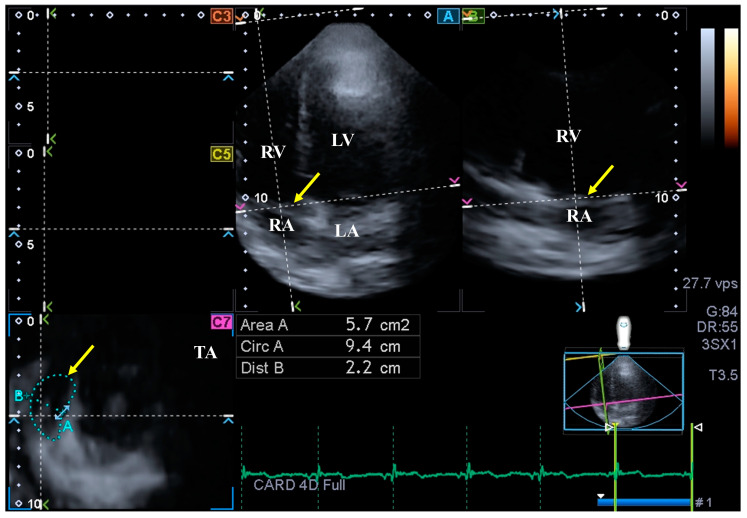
Extract from a three-dimensional full-volume dataset demonstrating the tricuspid annulus (TA) in an acromegaly patient: apical four-chamber (A) and two-chamber (B) views and a cross-sectional view at the TA level optimized in A and B views (C7). The yellow arrows represent the plane of TA. Abbreviations: LA = left atrium, LV = left ventricle, TA = tricuspid annulus, RA = right atrium, RV = right ventricle, Area = TA area, Circ = TA perimeter, Dist = TA diameter.
